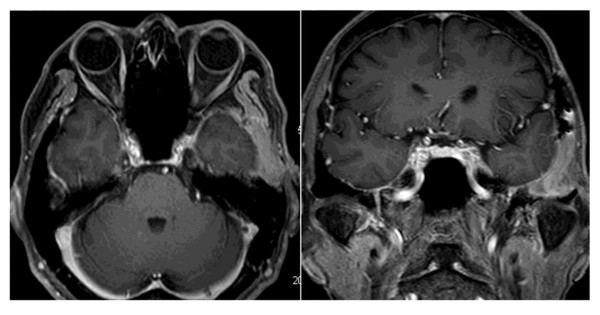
Magnetic resonance images with contrast one year after surgery reveal no enhanced region.
